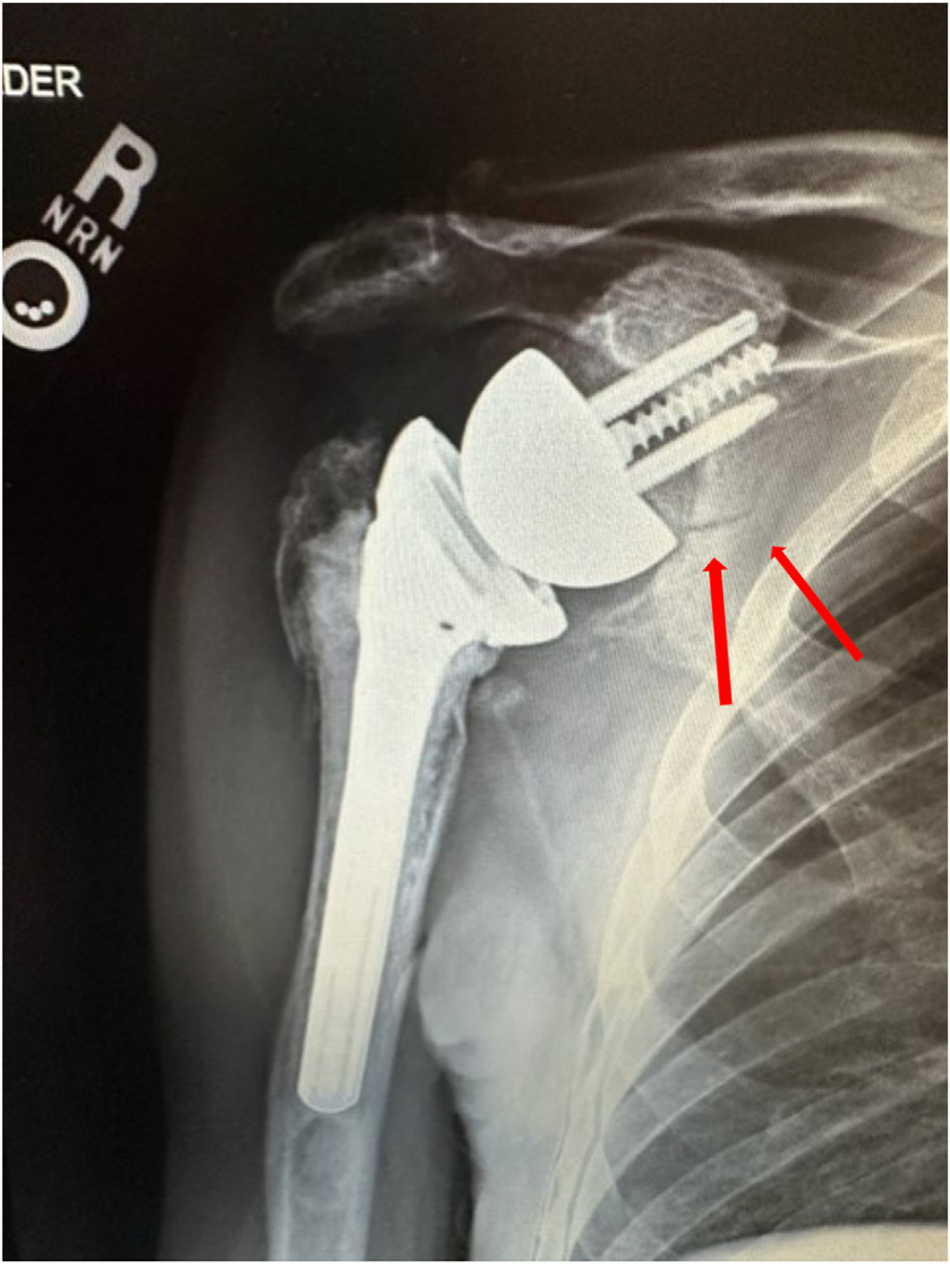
X-ray Grashey view demonstrating an incomplete fracture of the scapular body.
